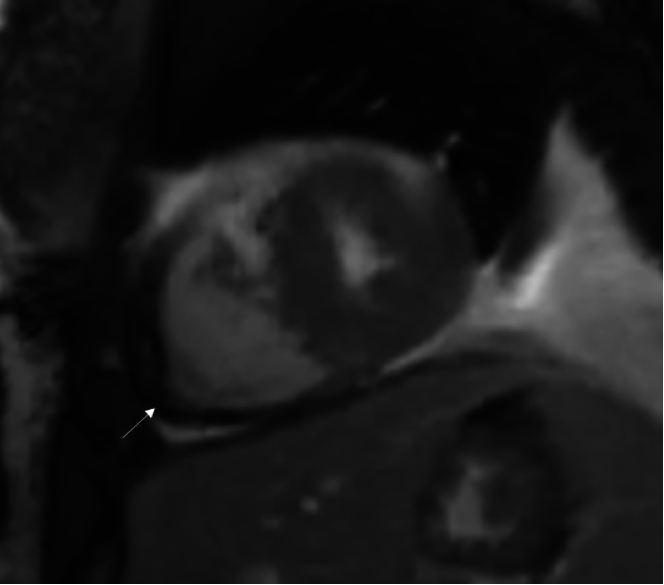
TrueFISP Cine Cardiac MRI in Short-Axis View There is a small aneurysm of the mid inferolateral right ventricular wall that demonstrates dyskinetic motion on cine imaging. The arrow indicates the aneurysmal area. MRI = magnetic resonance imaging.
